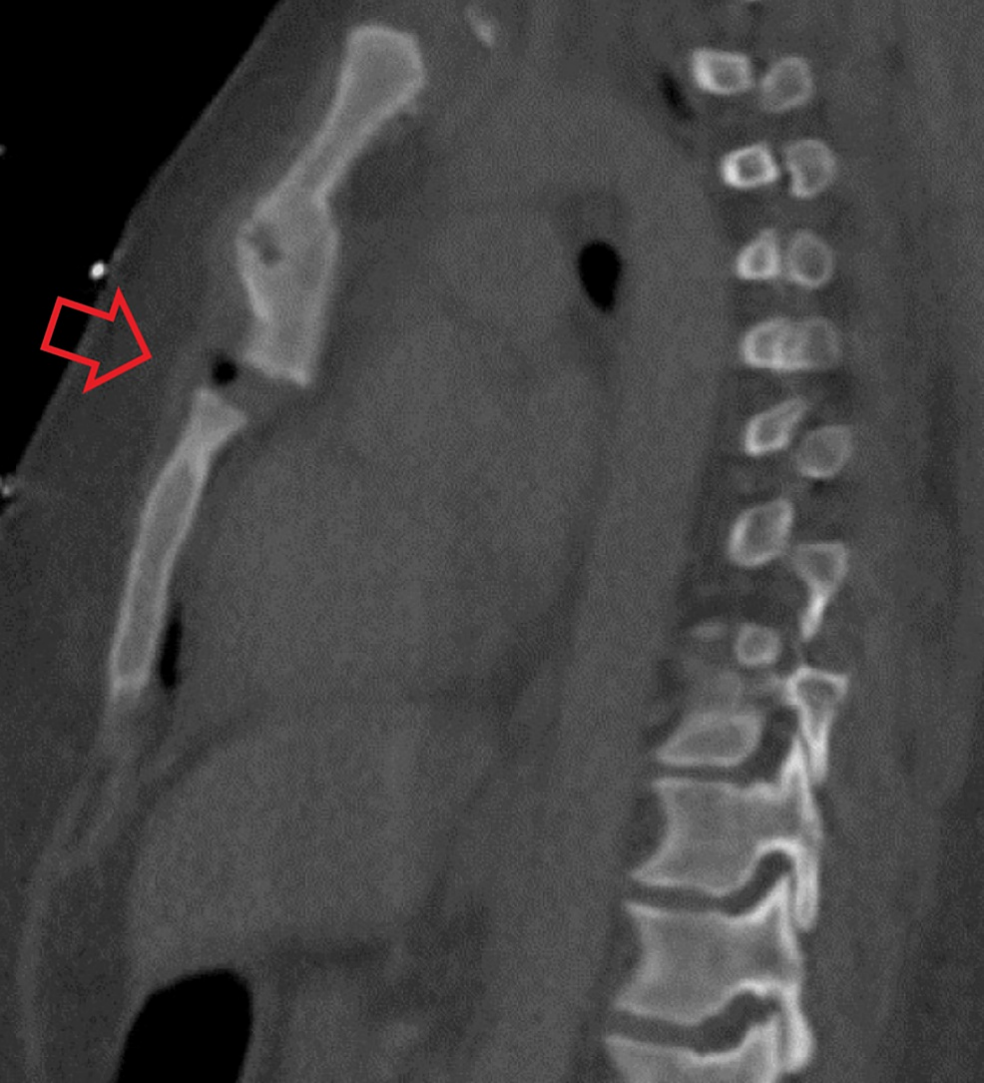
Sagittal CT image demonstrating posteriorly displaced and superiorly distracted sternal fracture (bone window). The red arrow points to the location of the fractured sternum.
